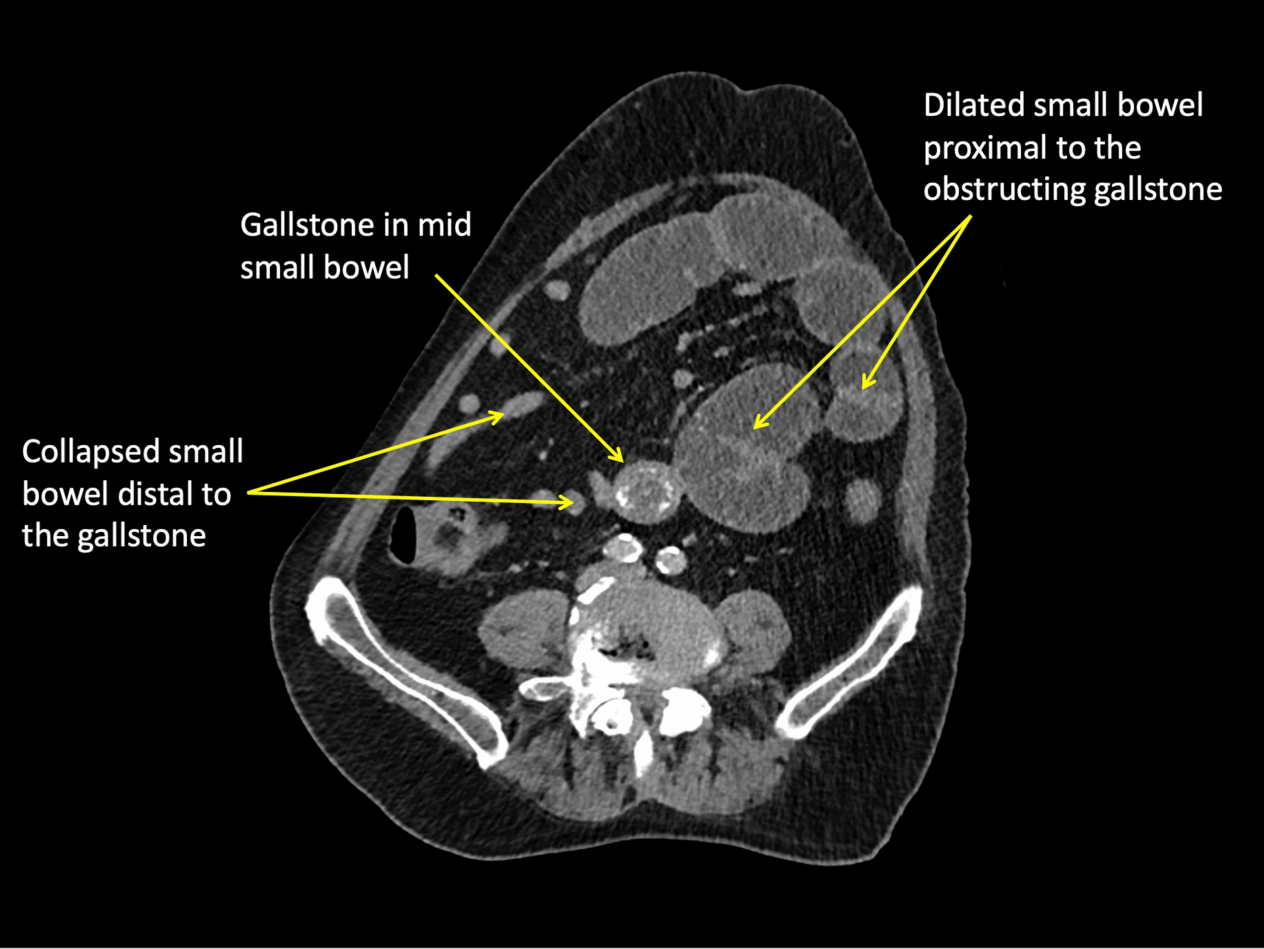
Axial CT showing gall stone in mid small bowel causing proximal small bowel dilatation and collapsed distal loops.
